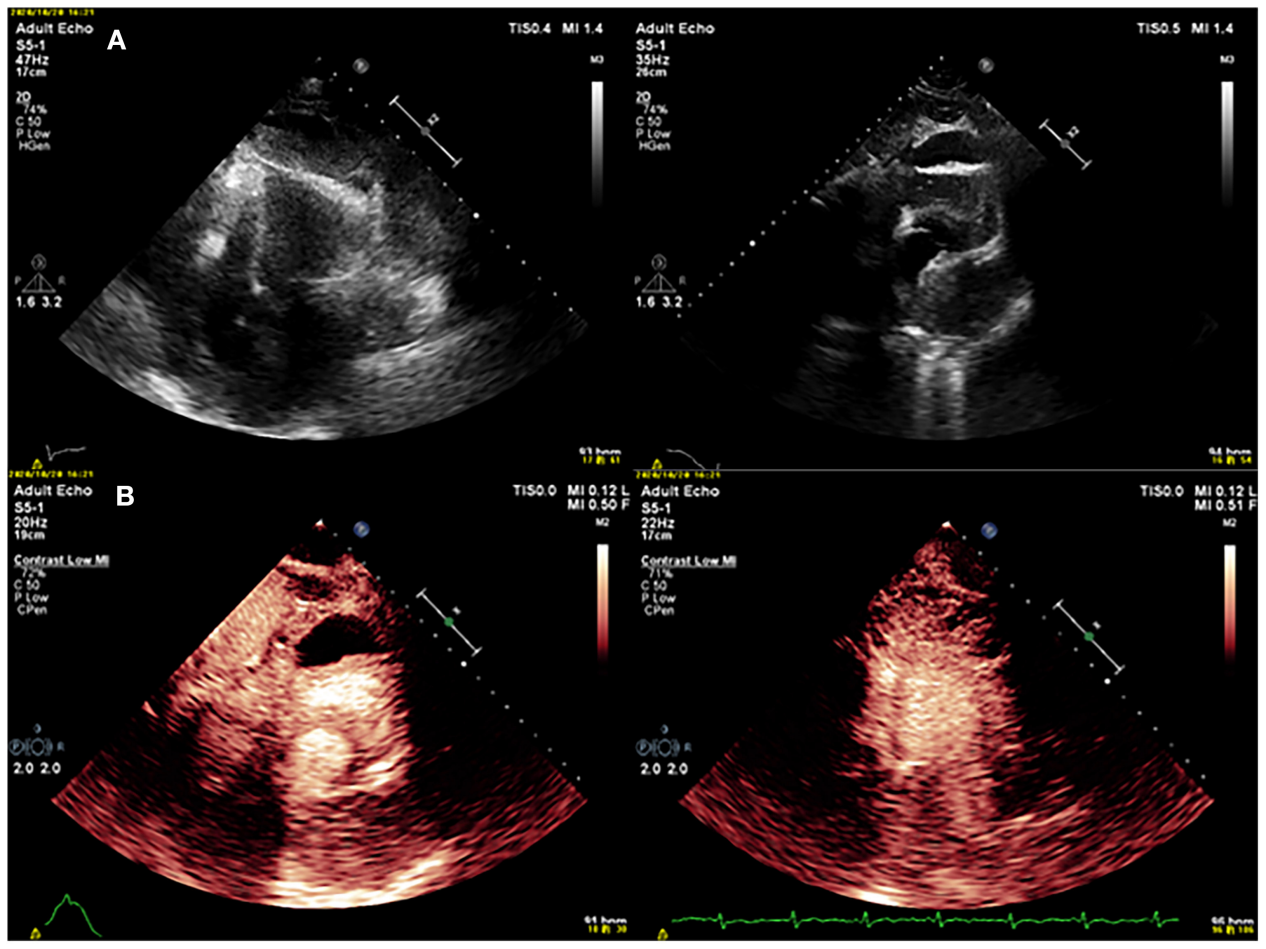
(A) Conventional echocardiography showed a mass in the pericardium. (B) Contrast echocardiography showed that the mass was hyper-enhancing.
